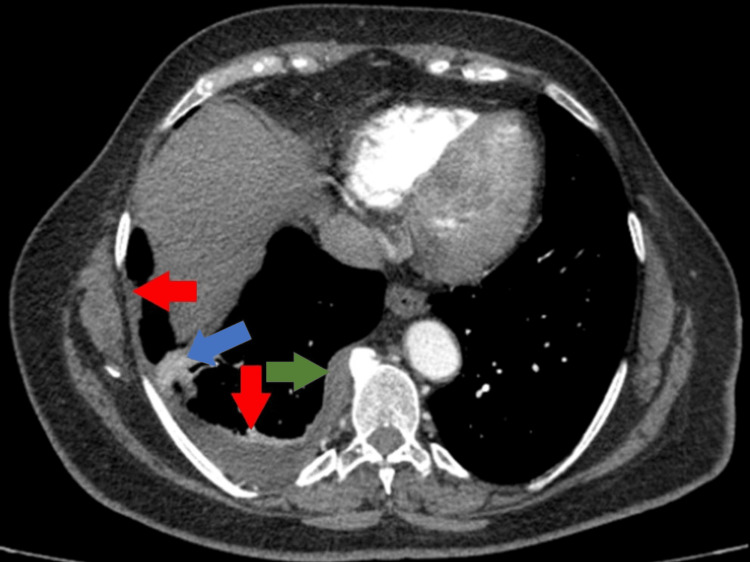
Contrast chest CT scan (mediastinal window, axial plane) showing recurred pleural effusion, pleural fluid collections, subpleural lamellar pulmonary consolidation and pleural thickening
